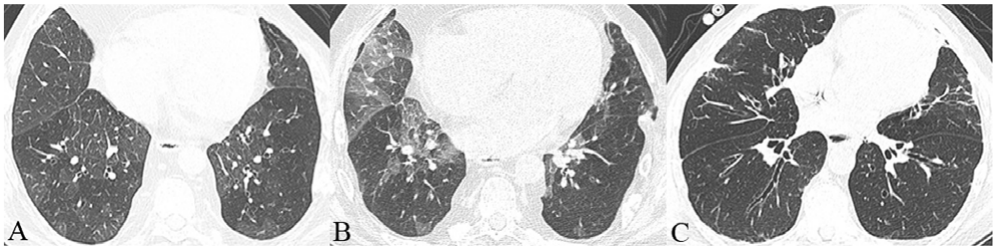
Typical CT findings of BOS include bronchial wall thickening (A), mosaic attenuation (A), air trapping (B) and bronchiectasis (C).
